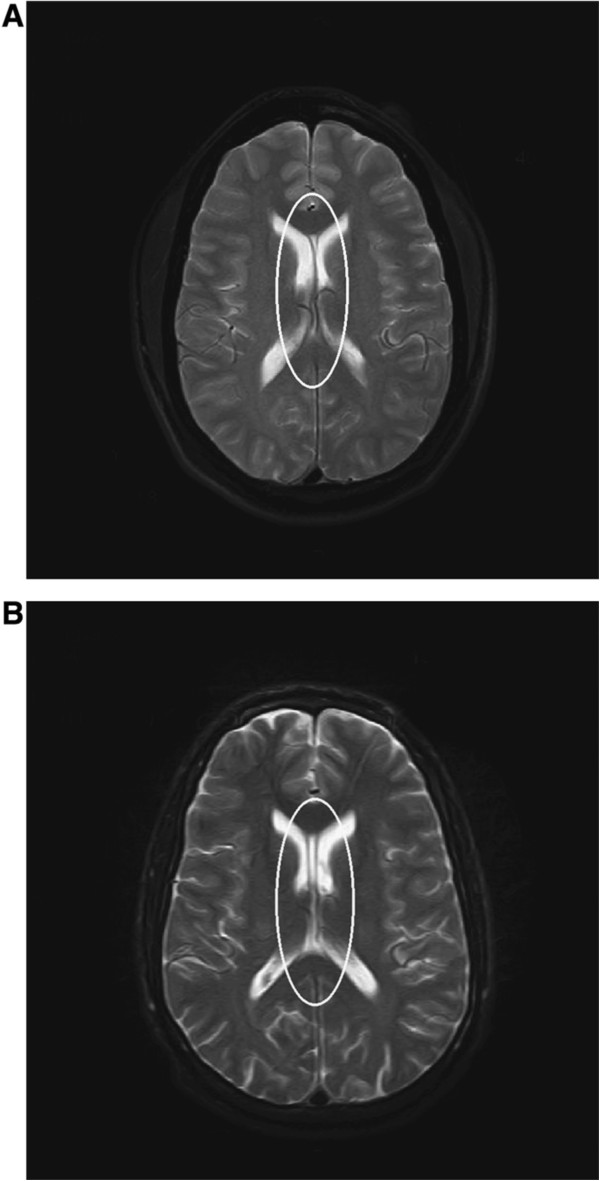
Cavum septum pellucidum (CSP) on MRI. (A) Mild (B) Moderate. No severe CSP was observed. Orrison et al. [107] examined one hundred consecutive unselected MRI scans that were performed on professional unarmed combatants (boxers and mixed martial arts fighters) in two outpatient imaging settings. Seventy-five were imaged on a 1.5-Tesla (T) MRI system and 25 were imaged on a 3.0-T high field MRI system. CSP was defined as the presence of a fluid filled space separating laminae of the septum pellucidum. CSP was graded as mild, moderate, or severe. CSP was found in 42% of subjects, due in part due to the improved resolution of the higher field strength MRI systems used.
